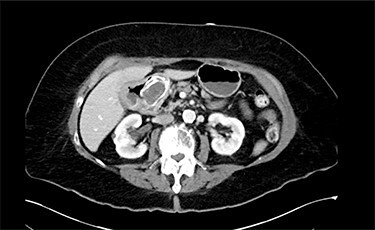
Abdominal CT scan (axial plane) showing gallstone in the duodenum with a cholecystoduodenal fistula.
